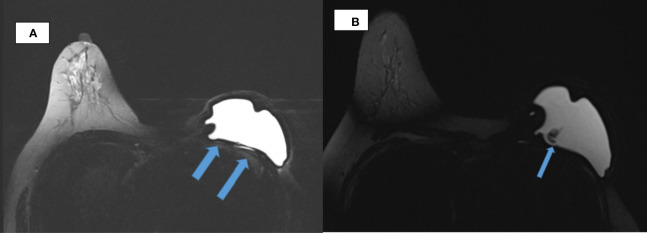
Breast MRI. (A) Axial TIRM image of MR breasts demonstrates irregularity of the silicone implant shell with inward invagination in the left breast. Linear hyperintensity that is of fluid intensity noted posterior to the implant till its lateral border raises the suspicion of an extracapsular rupture (arrowed). (B) Wavy lines (arrowed) evident within the silicone implant giving the linguine sign, which suggest an intracapsular rupture.
